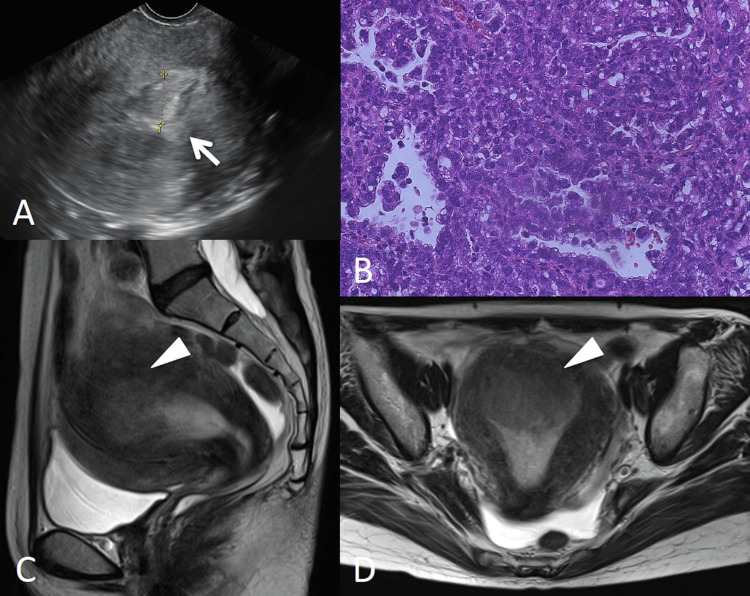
Vaginal ultrasonography shows endometrial thickening (arrow) (A). Endometrial sampling demonstrates a grade 2 endometrioid carcinoma (B). T2-weighted magnetic resonance image of the pelvis reveals a 6‑cm intrauterine tumor (C, sagittal section; D, axial section; arrowhead) without pelvic lymph node enlargement.
